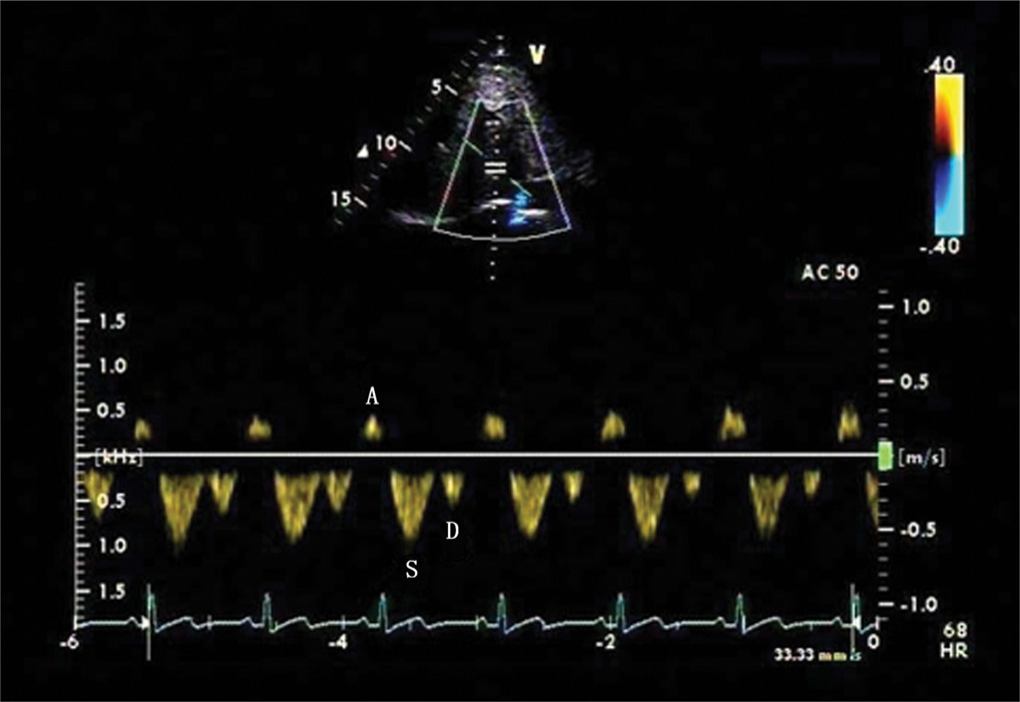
Tri-phasic Doppler flow spectra of the middle hepatic vein (MHV) in the control group. Doppler spectra of MHV obtained from a patient with atrial septal defect with the mean pulmonary artery pressure (MPAP) of 13 mmHg. The tri-phasic waveform includes ventricular systolic (S wave), ventricular diastolic (D wave) and atrial reversal (A wave) waves.
